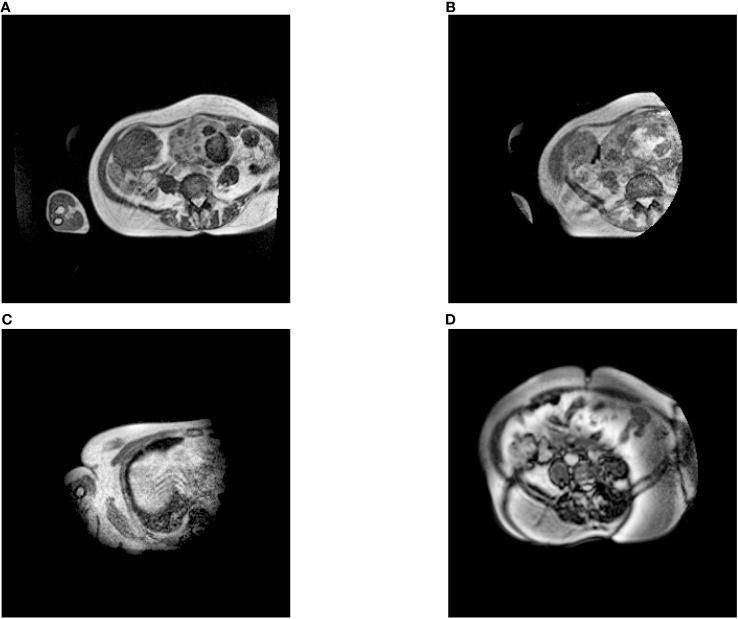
Examples of axial MR images from different exams. (A) is the image we kept, and (B–D) were removed. Specifically, (B, C) are only half exposed, while half of (D) is not clear.
